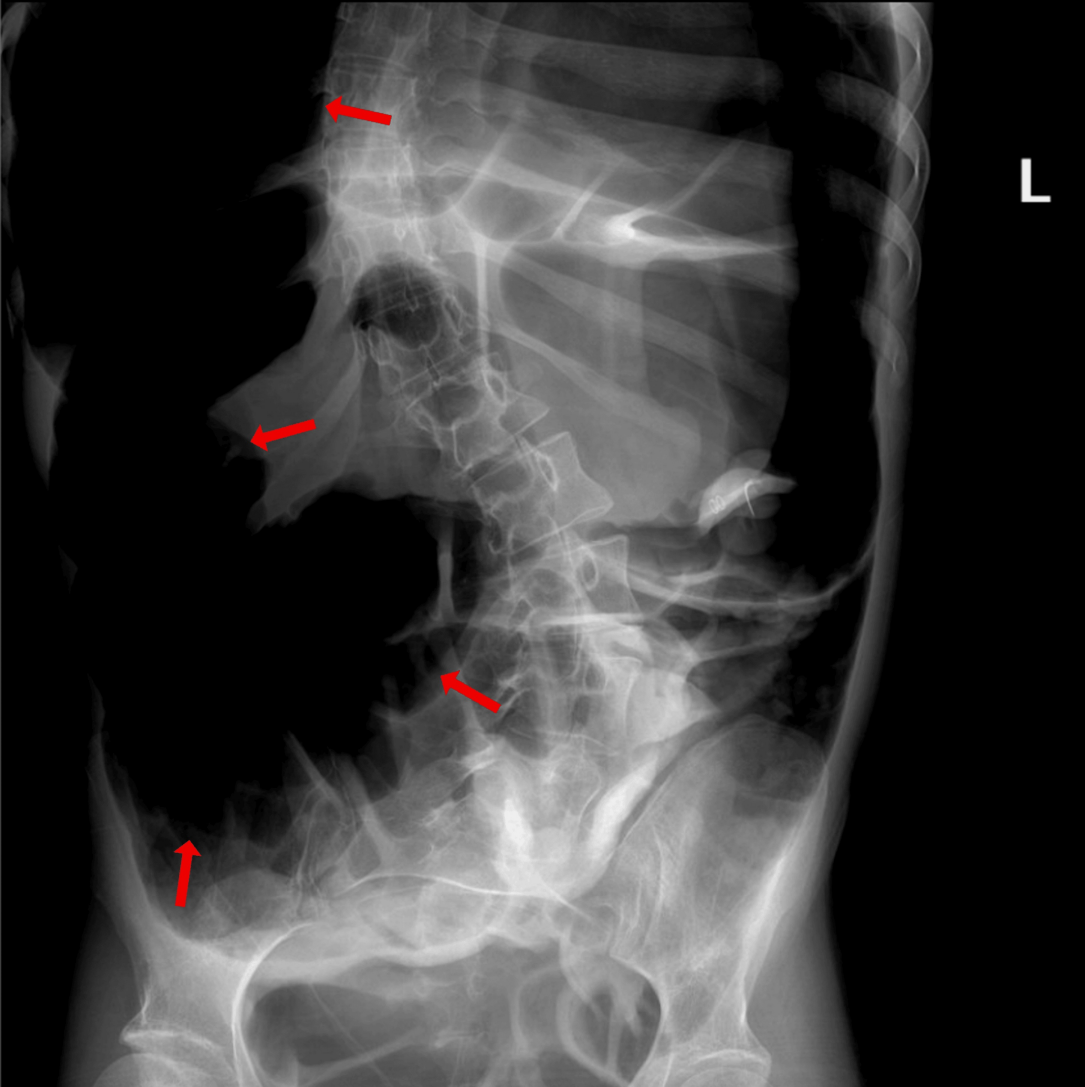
Initial abdominal X-ray demonstrating diffuse gaseous bowel distention with possible megacolon Supine anteroposterior abdominal radiograph from the initial emergency department visit shows widespread gas throughout the small and large bowel (arrows). The colon reaches a maximal diameter of approximately 8 cm, raising concern for ileus or megacolon. No fecal loading or free intraperitoneal air is seen.
